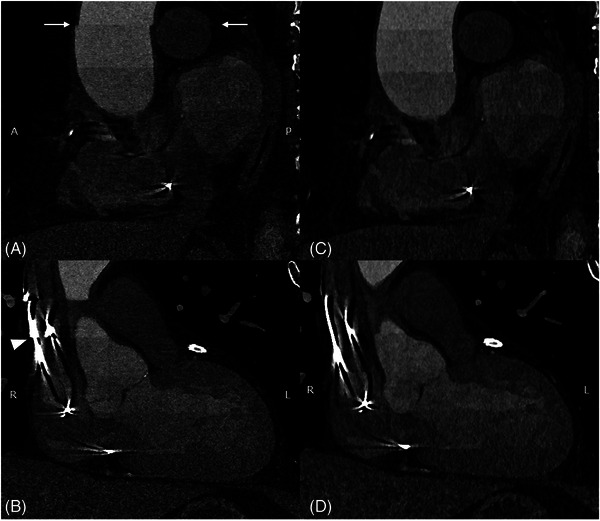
Sagittal (A) and coronal (B) multiplanar reconstructions of an 80‐year‐old male using UHR PCD‐CT (0.2 mm slice thickness, quantum iterative reconstruction strength level of 4, window level 300, width 900). On the sagittal view, a stairstep artifact can be seen affecting the ascending aorta and the right pulmonary artery (arrows). On the coronal views, the artifact can be readily observed affecting the partly imaged pacemaker leads (arrowhead). After the application of a special cardiac stack correction software, proper alignment has been restored on both sagittal (C) and coronal (D) views.
